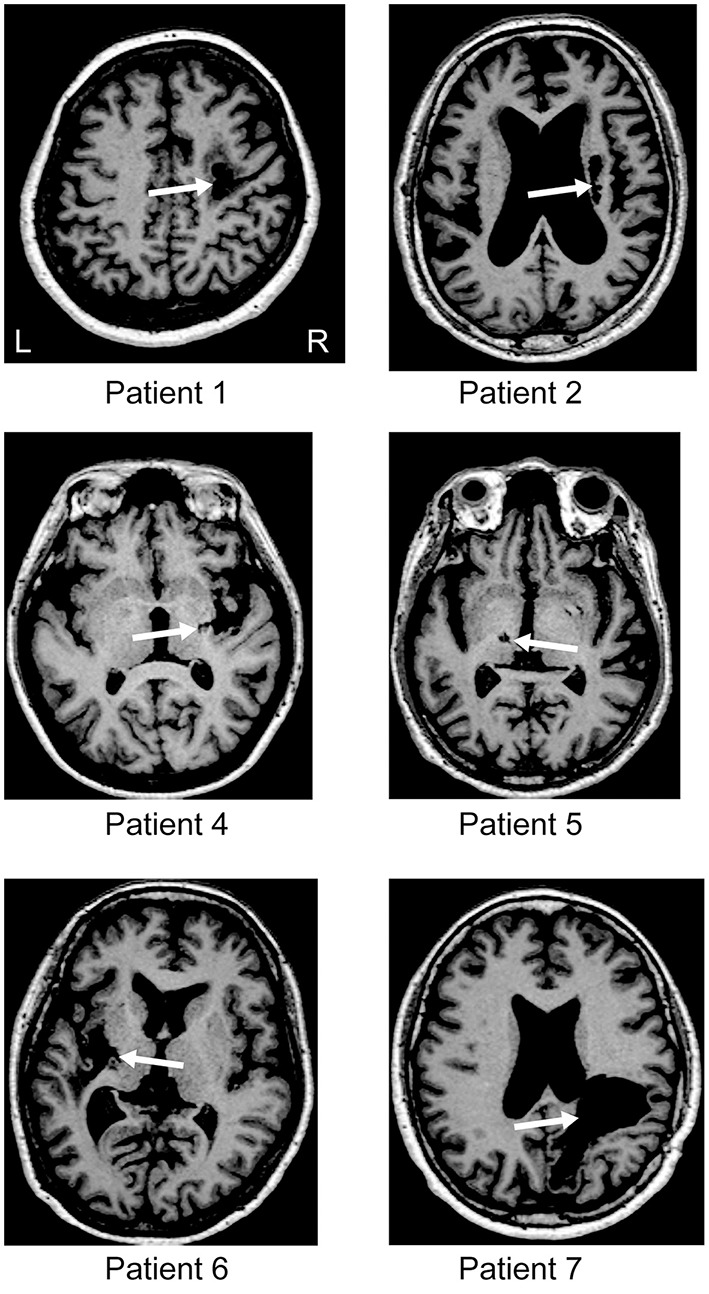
Brain imaging. T1 magnetic resonance imaging (MRI) at the level of the main stroke for each patient. For patients 3 and 8, the MRI data were missing. White arrows indicate the location of the lesion. L and R represent the left and right hemisphere, respectively.
